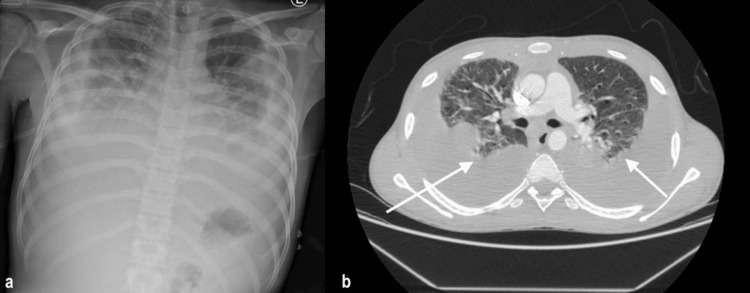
Initial images on presentation (a). X-ray showing massive bilateral effusion. (b) CT scan showing massive bilateral effusion with atelectatic changes bilaterally (white arrow).
